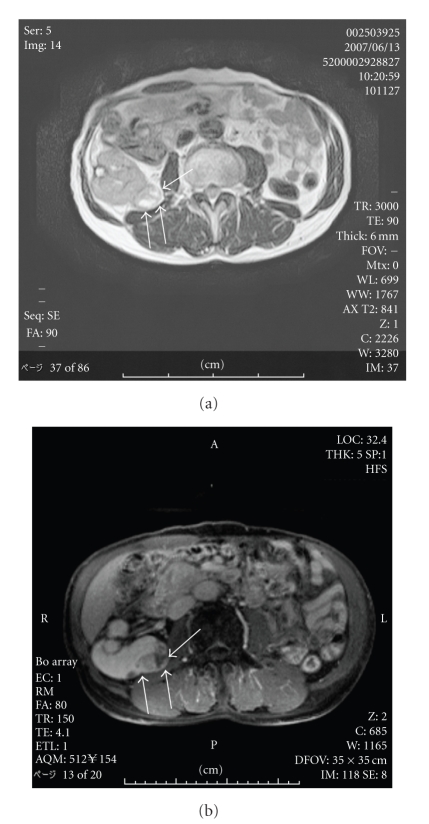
(a) T1-weighted magnetic resonance imaging showed a solid mass measuring 3 cm with heterogeneous intensity in the residual right kidney (case number 4). (b) The local tumor was well-controlled for 20 months after CT-guided RFA.
